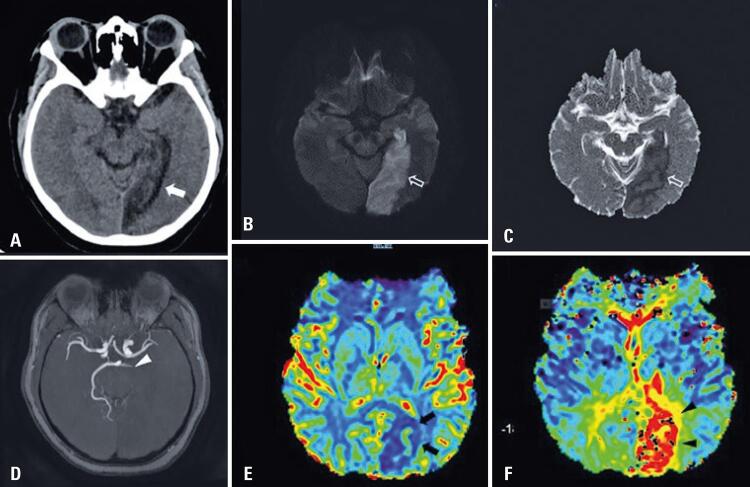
Computed tomography of patient 2. (A) Subacute ischemic lesion involving the left temporal and occipital lobes (white arrow). Magnetic resonance imaging (B and C) showing true restriction, which is seen in the diffusion images and in the apparent diffusion coefficient (empty arrows). Magnetic resonance angiography (D): left posterior cerebral artery occlusion (white arrowhead). Perfusion study (E and F): reduced relative cerebral blood volume (black arrows) and increased mean transit time (black arrowhead) seen in the area corresponding to the ischemic lesion, without significant penumbra
